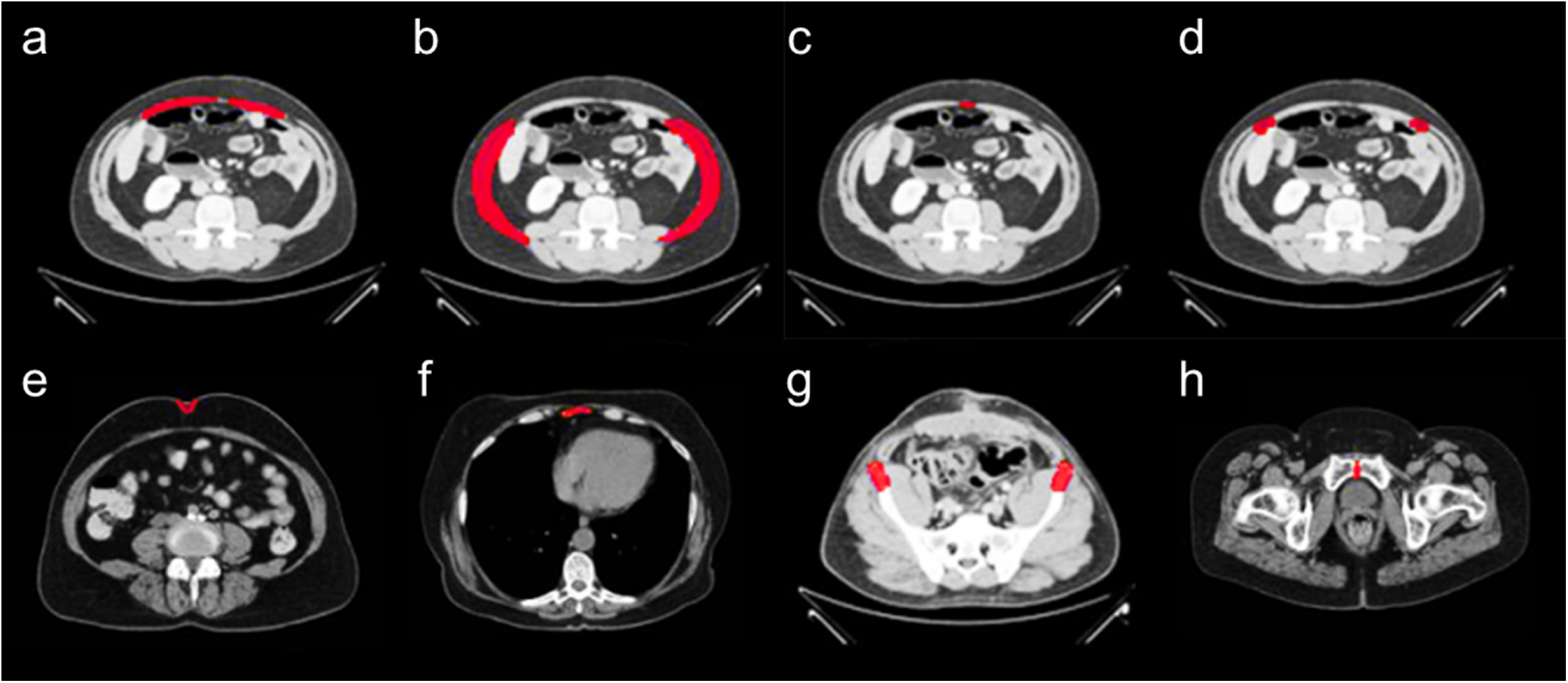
Anatomical structures included in the CT labeling protocol. (a) rectus muscles; (b) oblique abdominal muscles; (c) linea alba; (d) linea semilunaris; (e) umbilicus; (f) xiphoid process; (g) anterior superior iliac spines; and (h) pubic symphysis.
